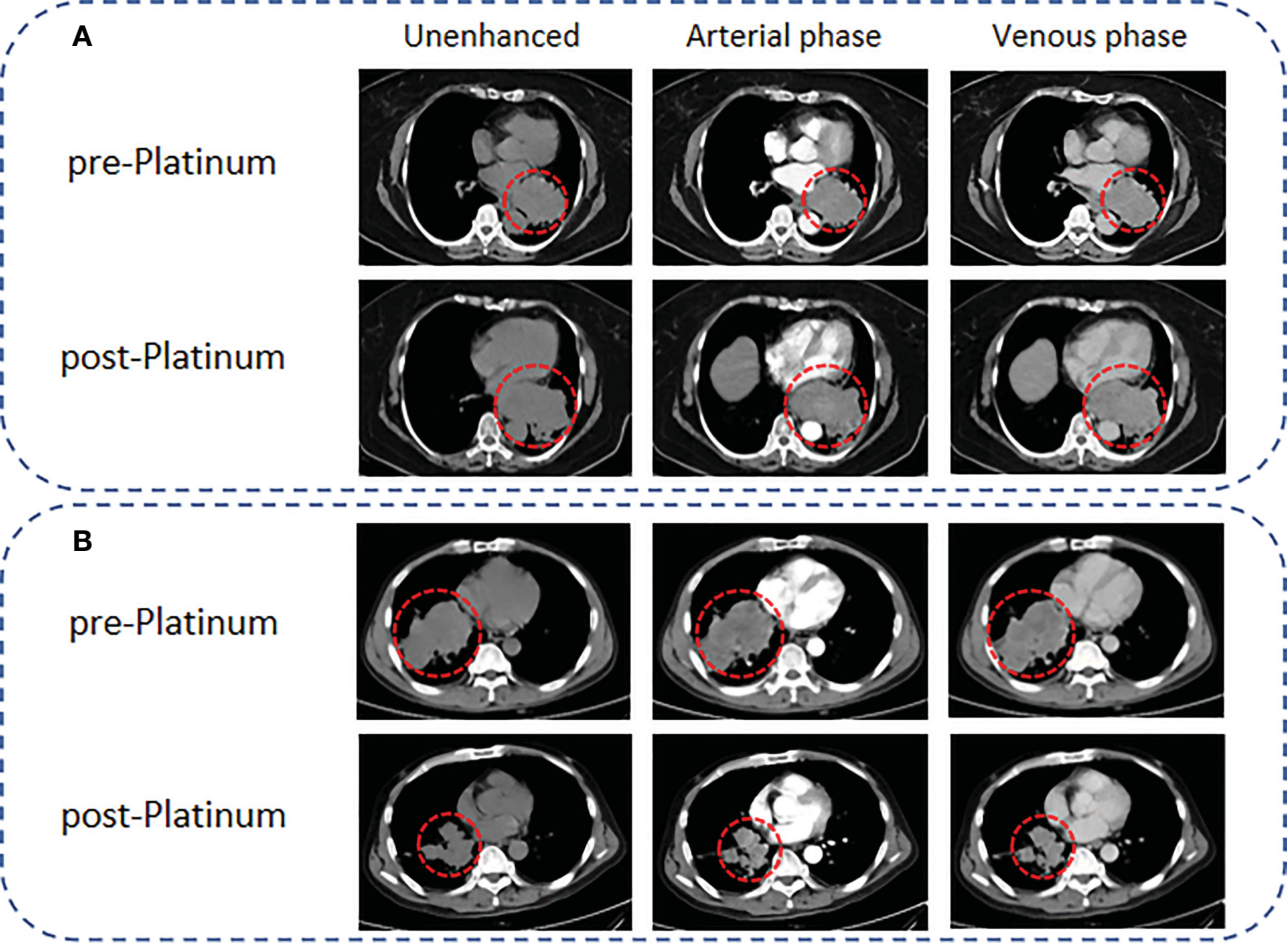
Representative CT images for PR and PS of SCLC patients according to the mRECIST criteria. (A) A 65-year-old female SCLC patient with a lesion diameter of 71 mm underwent CT scanning 1 week before EP chemotherapy, followed by CT scanning 2 months later. The lesion diameter increased to 97 mm, and the results showed that the patient presented with PR. (B) A 54-year-old male SCLC patient with a lesion diameter of 95 mm. CT scanning was performed 1 week before EP chemotherapy, and follow-up CT examinations were performed regularly after EP chemotherapy. Ten months later, the lesion diameter decreased to 56 mm, and the results showed that the patient presented with PS.
